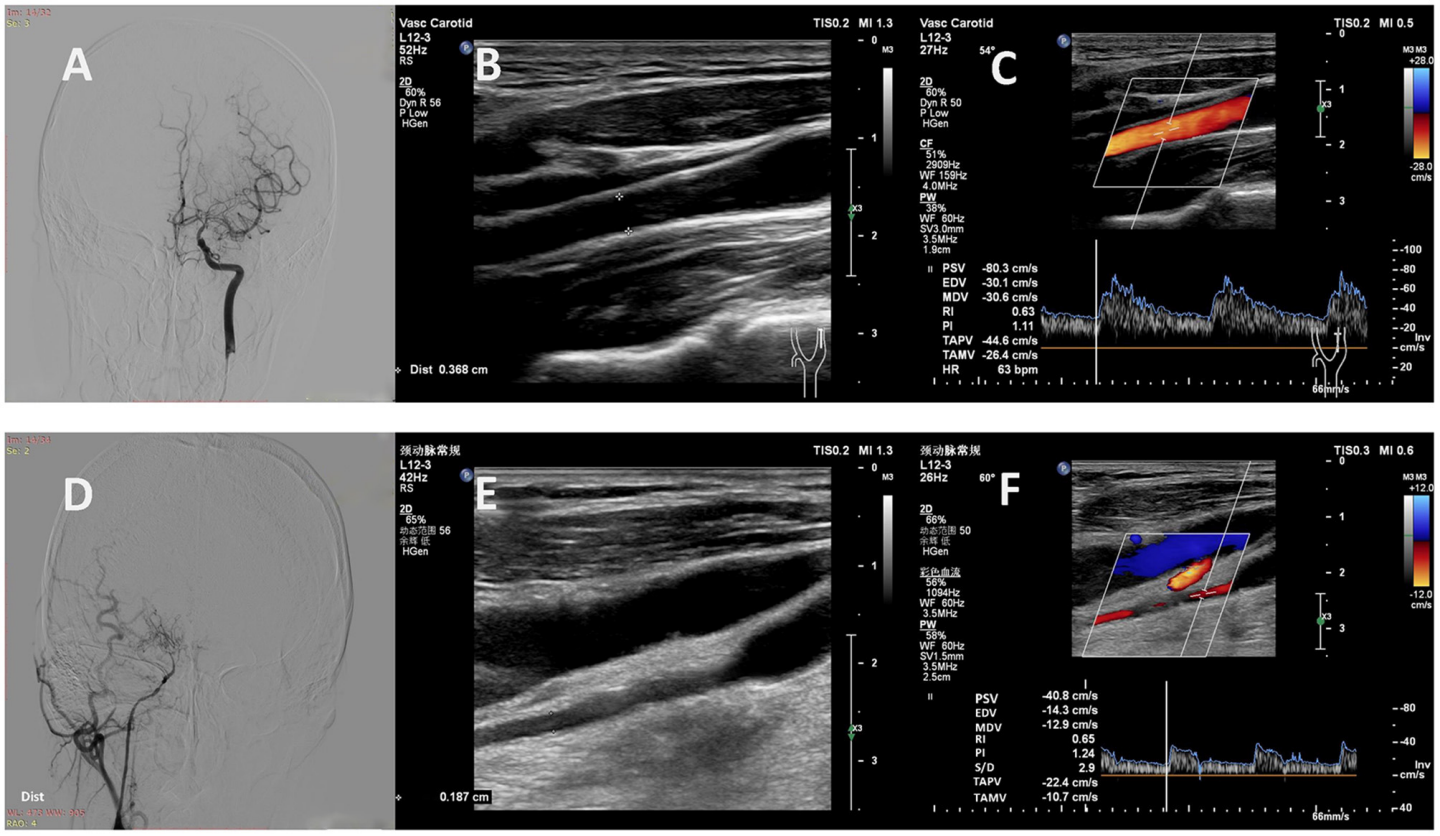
Representative cases. (A) A 32-year-old man with MMD had a Suzuki stage II on the left. (B, C) Carotid ultrasound showed high values of D (0.37 cm), PSV (80 cm/s), EDV (30 cm/s), TAMV (26 cm/s) and FV (168 ml/min) in the left EICA. (D) A 37-year-old woman with MMD had a Suzuki stage V on the right. (E, F) Carotid ultrasound showed low values of D (0.19 cm), PSV (41 cm/s), EDV (14 cm/s), TAMV (11 cm/s) and FV (18 ml/min) in the right EICA. MMD, moyamoya disease; D, diameter; PSV, peak systolic velocity; EDV, end diastolic velocity; TAMV, time-averaged mean velocity; FV, flow volume; EICA, extracranial internal carotid artery.
